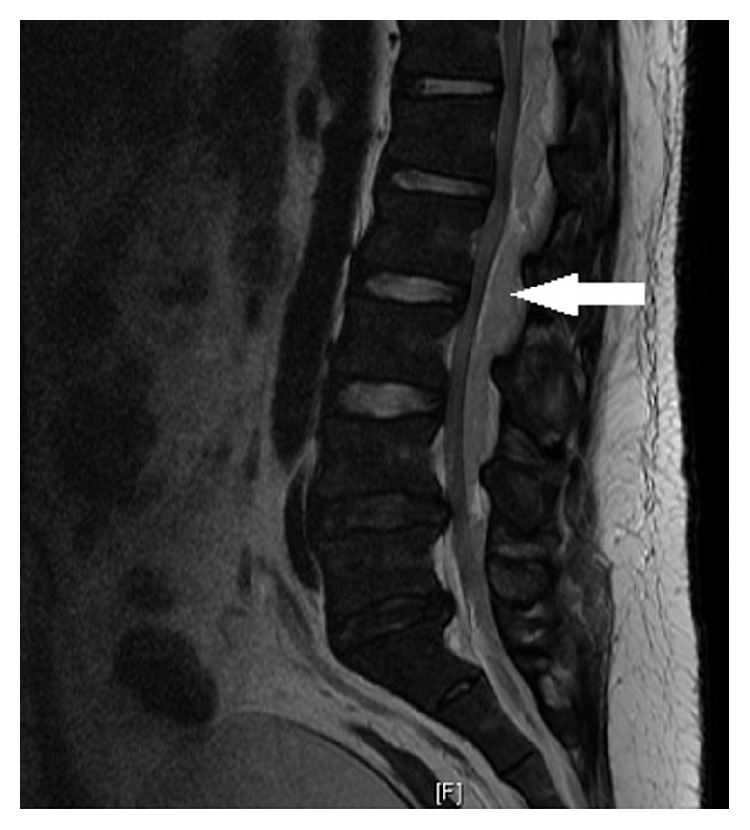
Sagittal view of T2 diffusion MRI lumbar spine showing extensive epidural abscess (arrow) extending from the upper dorsal region all the way to the distal conus medullaris, displacing the thecal sac.
